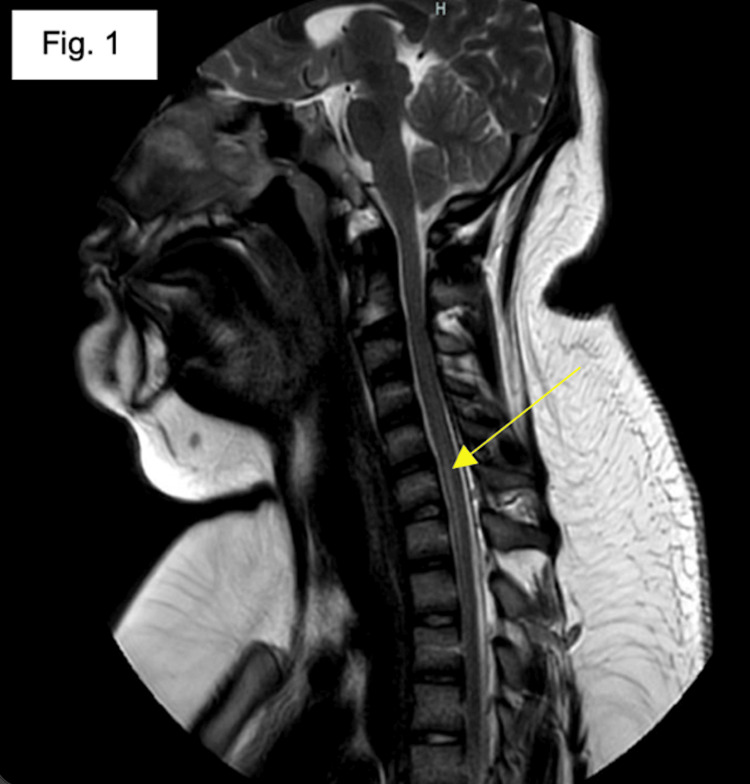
Sagittal T2-weighted cervical spine MRI showing intramedullary pencil-like hyperintensity extending from C5 to T3 (arrow).
